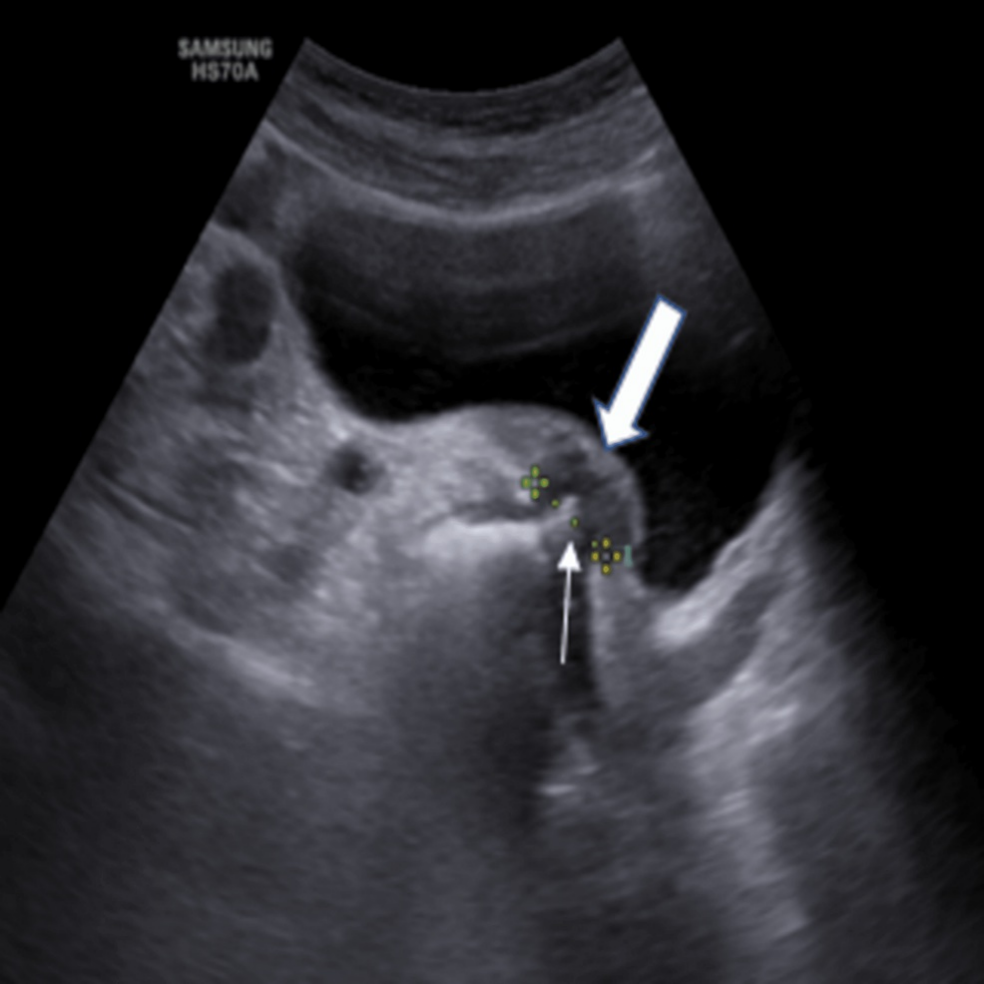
Ultrasonography axial section through the urinary bladder shows a fistulous connection between the sigmoid colon and urinary bladder (block arrow) through a defect (line arrow)
